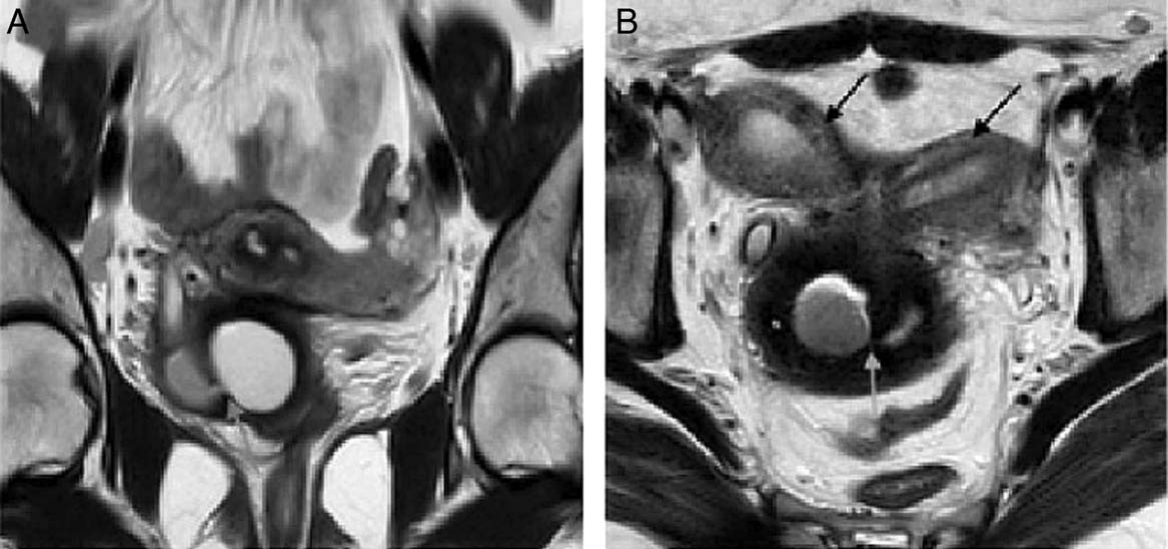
MRI T2-weighted coronal and axial image of a 23-year-old female showing a didelphic uterus (black arrow in 2B) and tiny communication between two hemivagina (white arrow in 2A and 2B).
